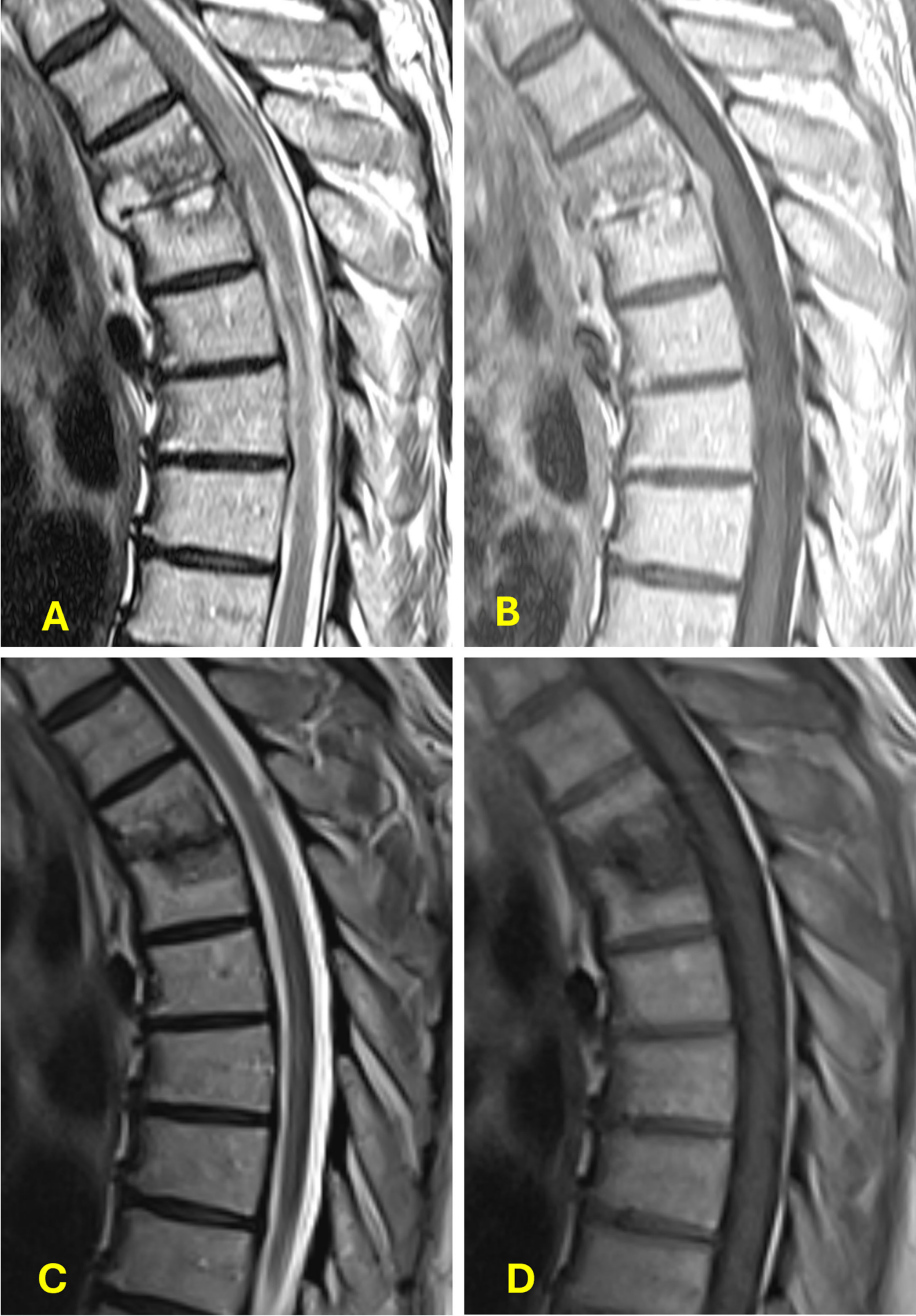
(A) T2-weighted image shows fluid signal in vertebral endplates and disc space, as well as anterior paraspinal. An intermediate density epidural soft tissue anterior to the cord without intrinsic fluid signal represents a phlegmon. (B) Contrast-enhanced T1-weighted image shows homogeneous enhancement of the anterior epidural phlegmon as well as patchy abnormal enhancement of the disc space and endplates. (C) T2-weighted image shows complete resolution of the anterior epidural phlegmon and resolution of abnormal fluid signal in vertebral endplates, disc space, and in the anterior paraspinal tissue. (D) An unenhanced T1-weighted image shows the resolution of the anterior epidural soft tissue.
